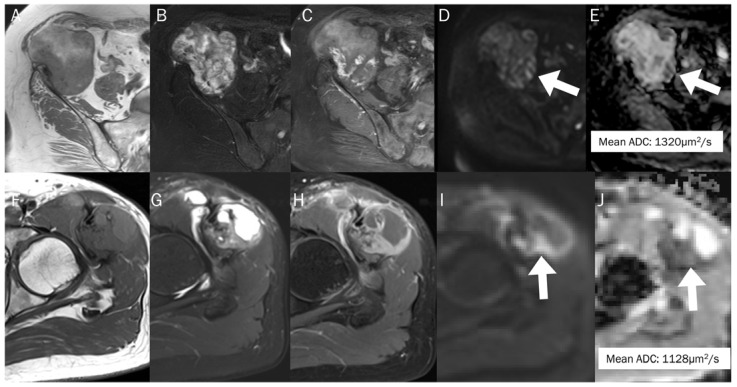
The differentiation between chronic expanding hematoma (A–E) and hemorrhagic malignant soft tissue tumor (F–J). Conventional axial MRIs of chronic expanding hematoma (A: T1-weighted, B: T2-weighted fat-suppressed, C: T1-weighted enhanced) show a lobulated mass with heterogeneously high T2 signal intensity and iso-to-high T1 signal intensity, located in the pelvic cavity, containing nodular enhancement. (D,E) DWI with *b*-value of 800 s/mm^2^ and ADC map exhibit an area of restricted diffusivity (arrow), and the mean ADC is 1320 μm^2^/s. Conventional axial MRIs of hemorrhagic high-grade sarcoma (F: T1-weighted, G: T2-weighted fat-suppressed, H: T1-weighted enhanced) show a lobulated mass with heterogeneously high T2 signal intensity and iso-to-high T1 signal intensity, located in the proximal thigh, containing nodular enhancement. (I,J) DWI with *b*-value of 800 s/mm^2^ and ADC map exhibit an area of restricted diffusivity (arrow) and the mean ADC is 1128 μm^2^/s.
